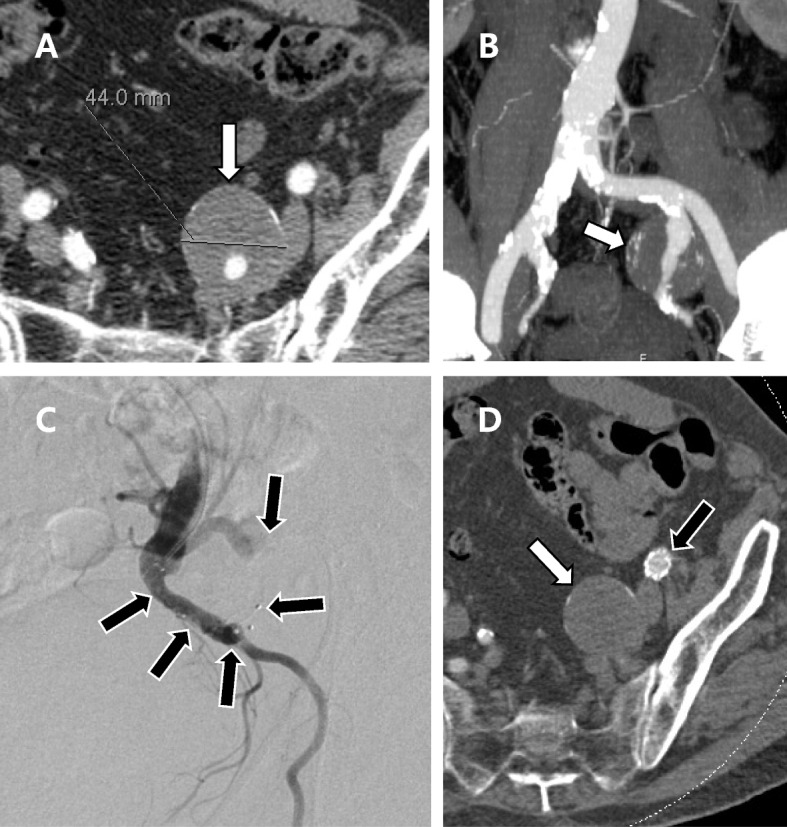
Successful endovascular treatment of an isolated IIA aneurysm in a 72-year-old, male patient. A and B CT scan demonstrates an IIA aneurysm measuring 44 mm in diameter (white arrow). C Vascular plugs (black arrows) were deployed in the outflow branches. The ostium of the internal iliac artery was covered by deploying an iliac stent graft (not shown). D CT at 3 months follow-up demonstrates complete thrombosis of the aneurysm sac (white arrow) without endoleak. Patency of the iliac stent-graft (black arrow) is maintained
